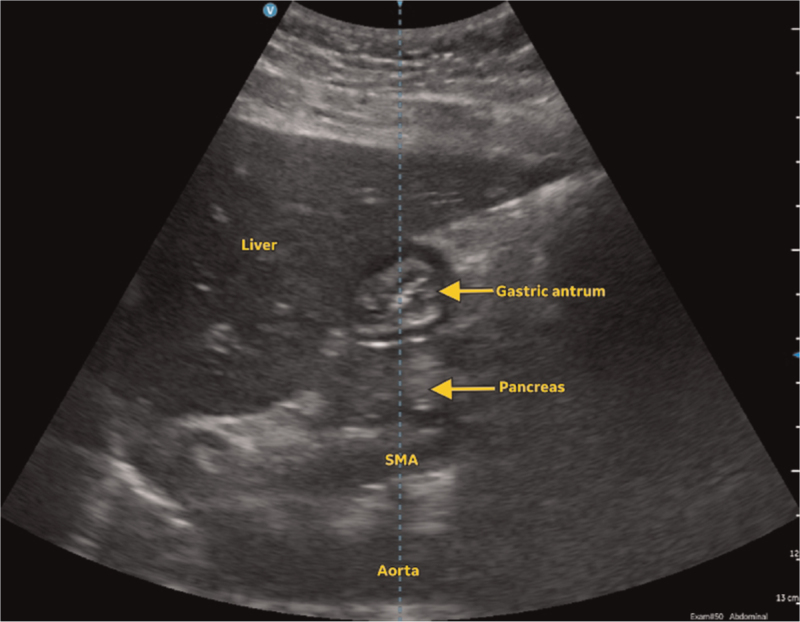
Ultrasound image of the gastric antrum when empty in a patient in the first trimester of pregnancy who has been fasted for 2 h for clear fluids and 6 h for solids.
